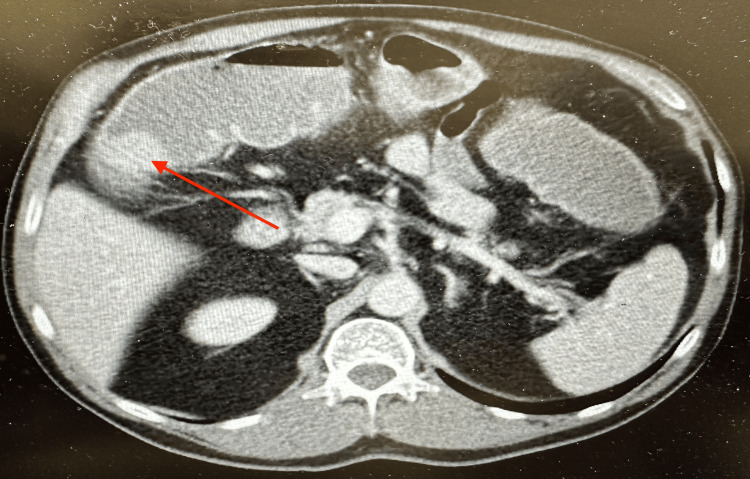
Contrast-enhanced computed tomography (CT) of the abdomen demonstrating circumferential wall thickening at the hepatic flexure. Axial CT image of the abdomen with intravenous contrast showing focal circumferential colonic wall thickening at the hepatic flexure (red arrow), resulting in luminal narrowing and proximal colonic dilation consistent with partial large bowel obstruction.
